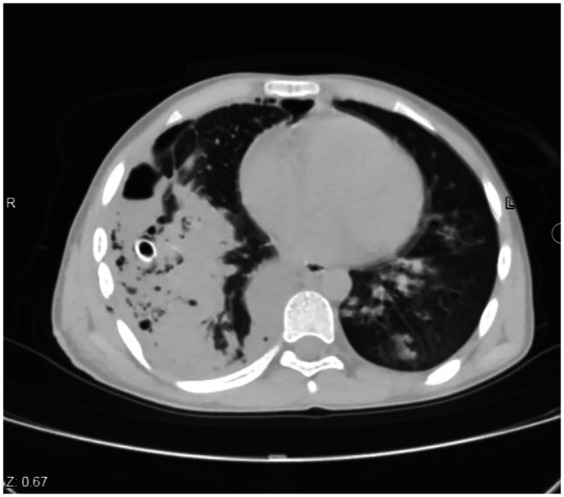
Computed tomography (CT) scan of the chest (axial section) 48 h after the intervention shows collapse consolidation involving the right lower lobe.
